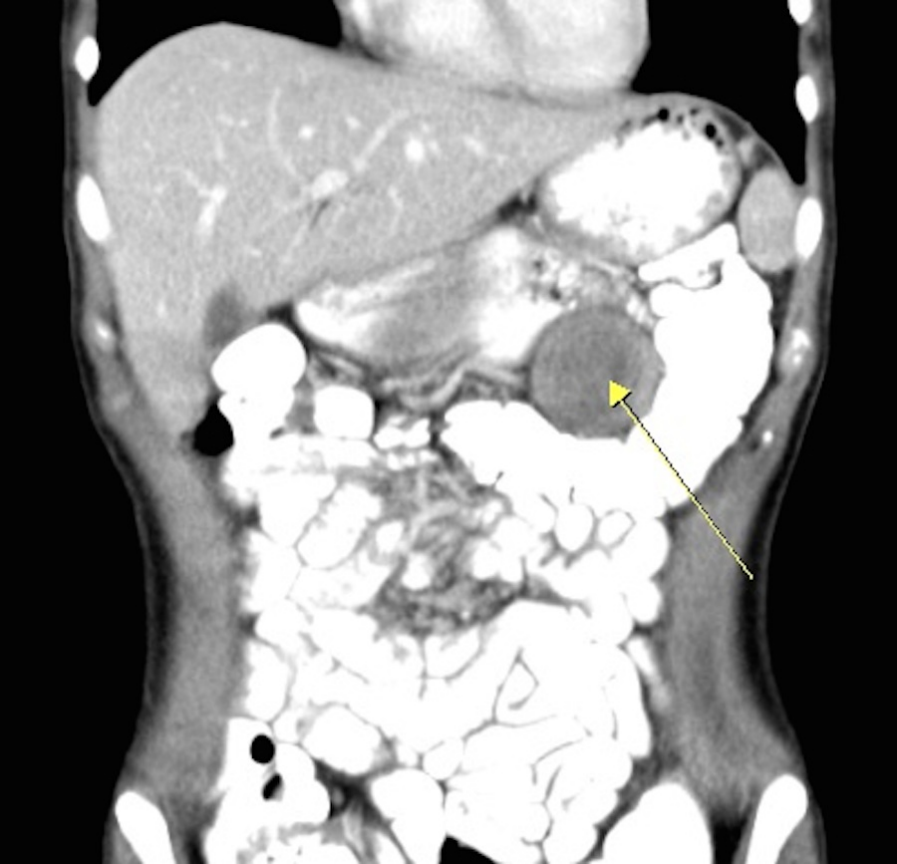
The Mass (arrow) in Coronal CT Plane
